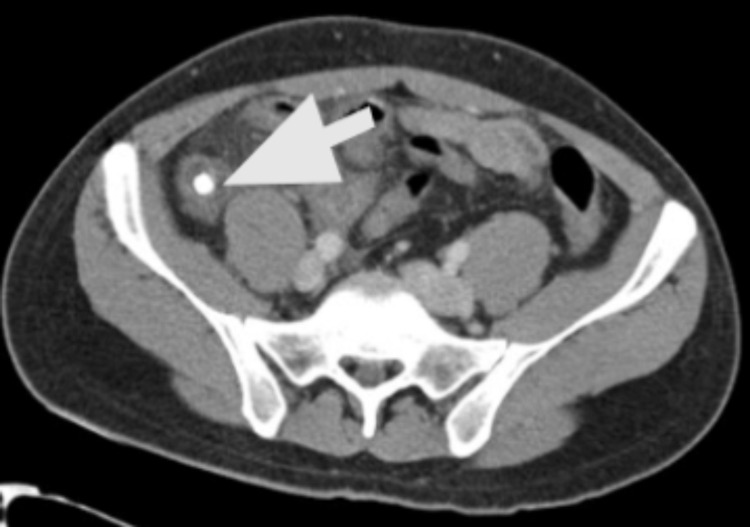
Contrast enhanced CT of the abdomen and pelvis showing 10 mm appendicolith at the base of the appendix
